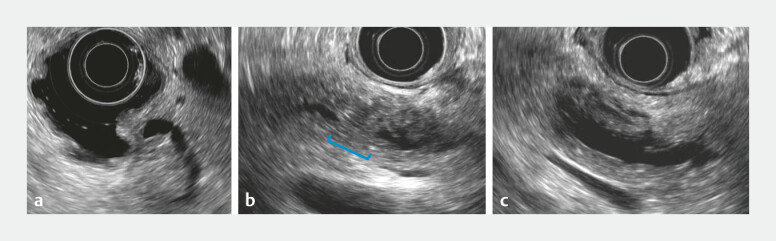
Endoscopic ultrasound (EUS) images.
